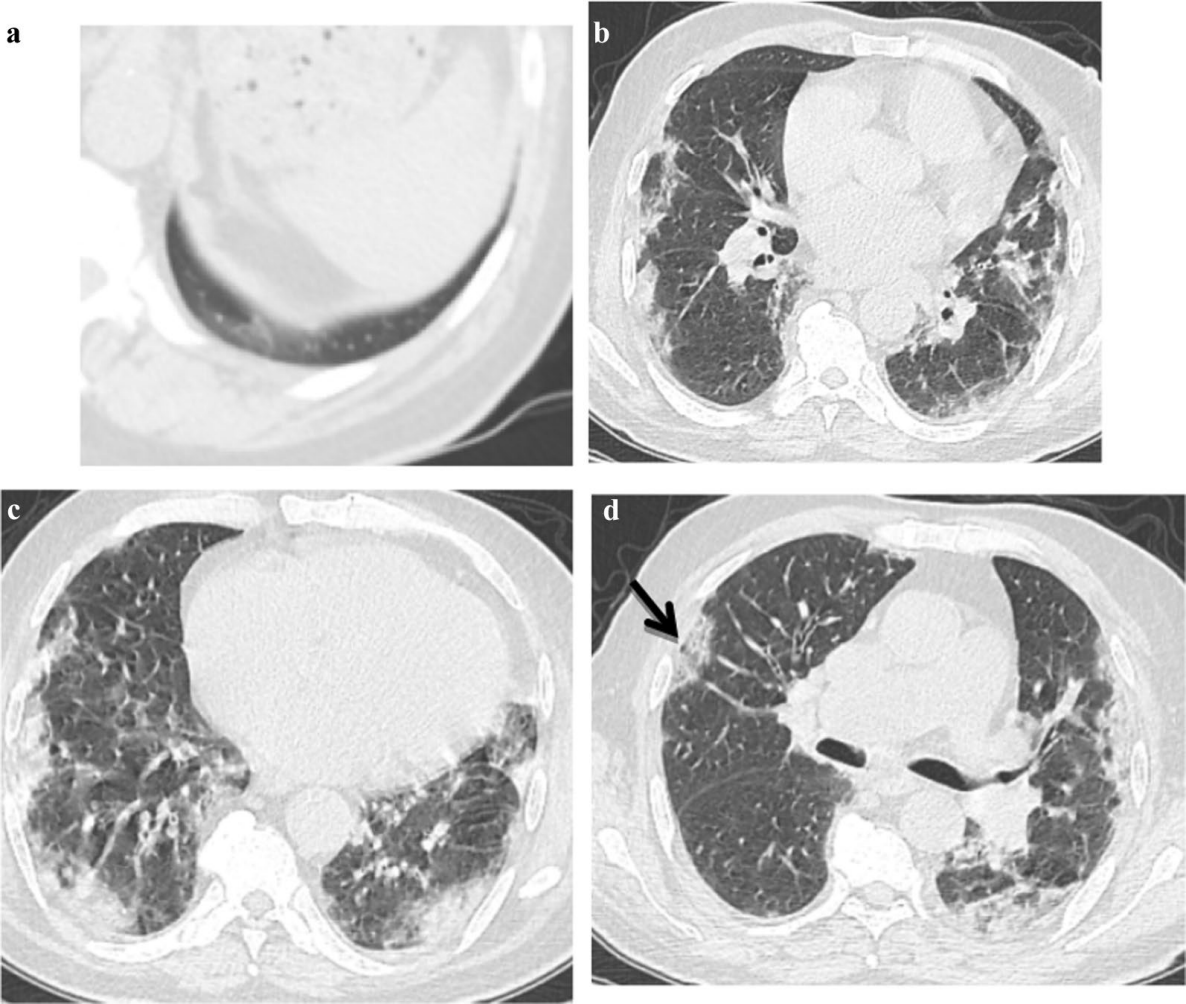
A 71-year-old male known cardiac presented with anosmia, fever and myalgia at March 2020 and positive PCR for COVID 19 infection, a noncontrast CT axial cuts shows bilateral few small subpleural faint ground glass opacities. The patient diagnosed as COVID 19 infection with CT score severity: 4. Second infection dated January 2021 the patient presented with fever, respiratory distress, cough and myalgia with positive PCR test for COVID 19 infection. b–d noncontrast CT chest images show bilateral patchy ground glass opacities with crazy paving sign (Arrowed) and consolidations. The patient diagnosed as COVID 19 re-infection with CT severity score: 18
